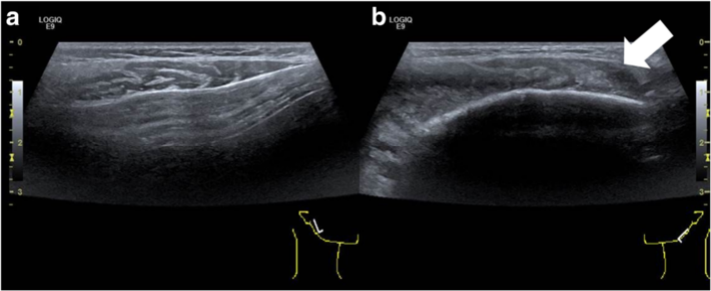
Ultrasound of the soft tissue of the cheeks (a, b). Inhomogenous widening on the left side (b) (*circle*)
